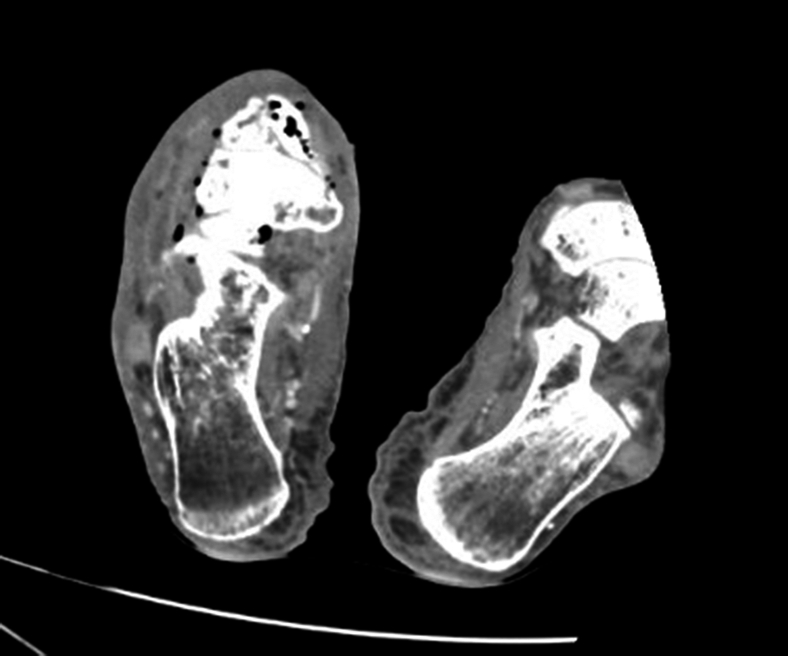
Axial computed tomography right lower extremity with air and stranding in deep tissues.
